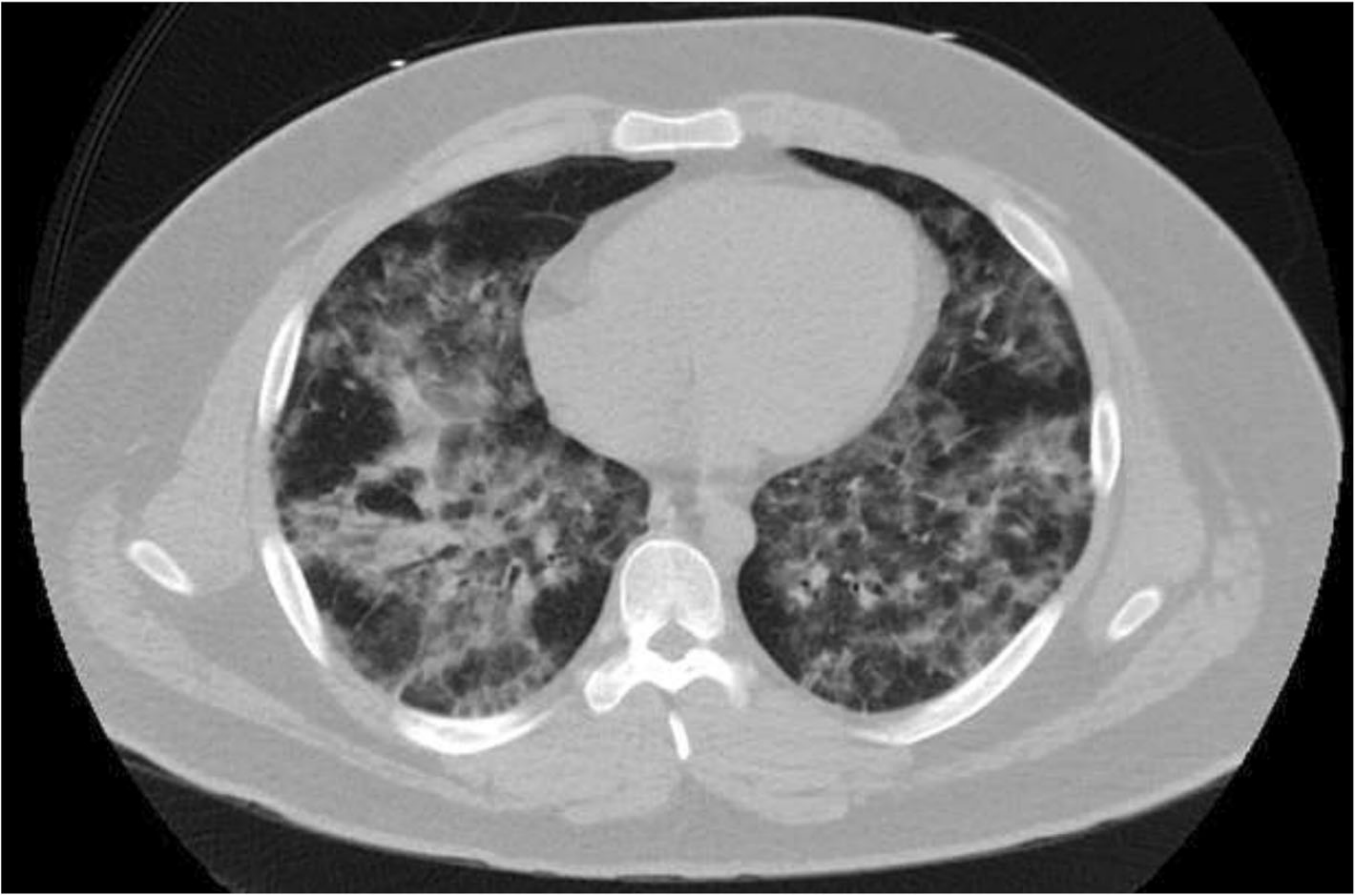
Axial CT Chest without IV Contrast: Diffuse interlobular septal thickening and ground-glass opacities involving the bilateral lung fields
